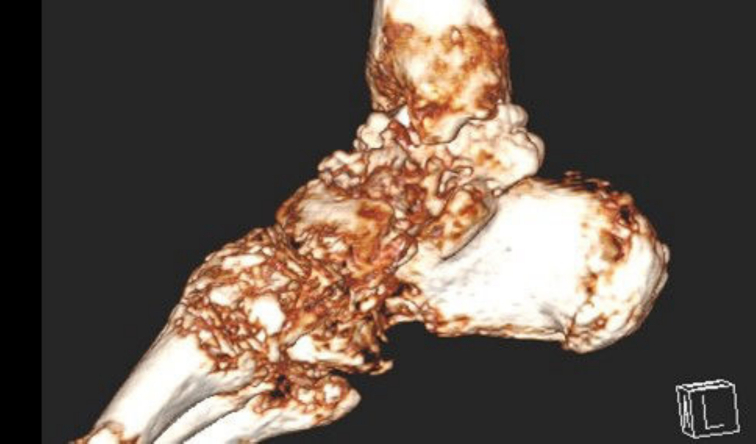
3D reconstruction CT scan of the ankle showing a lesion located in the right talus bone.
